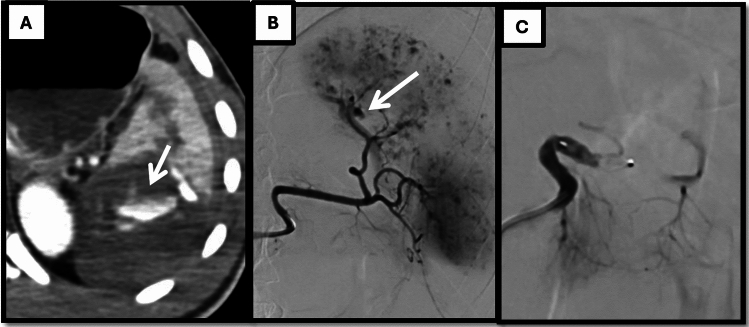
A Axial contrast-enhanced CT (CECT) of the abdomen in a 22-year-old male status post motor vehicle accident shows splenic laceration with active contrast extravasation (arrow). B Selective splenic arteriogram demonstrates a tortuous splenic artery with multiple pseudoaneurysms within the splenic parenchyma (arrow) C Post-embolization digital subtraction angiogram (DSA) with LOBO-7 shows successful proximal splenic artery occlusion with delayed opacification of the spleen via collateral vessels
